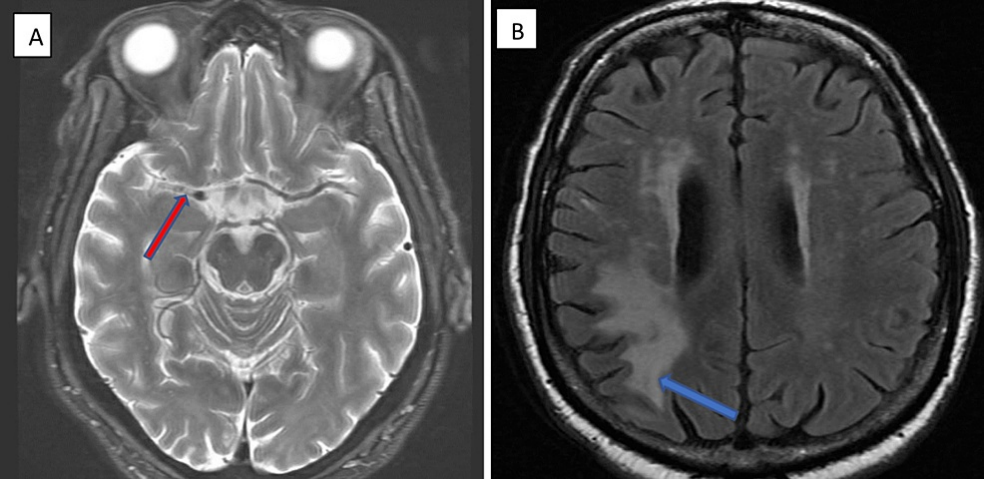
(A) T2 weighted axial MRI shows an absence of signal void in the right middle cerebral artery (red arrow). (B) T2 fluid-attenuated inversion recovery image shows chronic infarct with encephalomalacia involving the right posterior parietal lobe (blue arrow).
